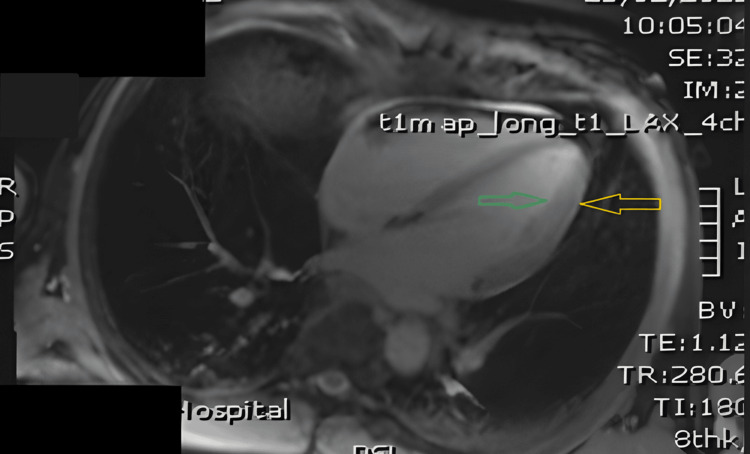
Cardiac MRI demonstrating preserved left ventricular size and systolic function. Long-axis, four-chamber, T1-mapping, cardiac MRI demonstrating normal biventricular size and morphology, with uniform myocardial signal and no evidence of edema or fibrosis. The left ventricular myocardium (yellow arrow) and endocardium (green arrow) appear normal. No pericardial effusion is present, and the atria are normal in size with no structural abnormalities identified.
